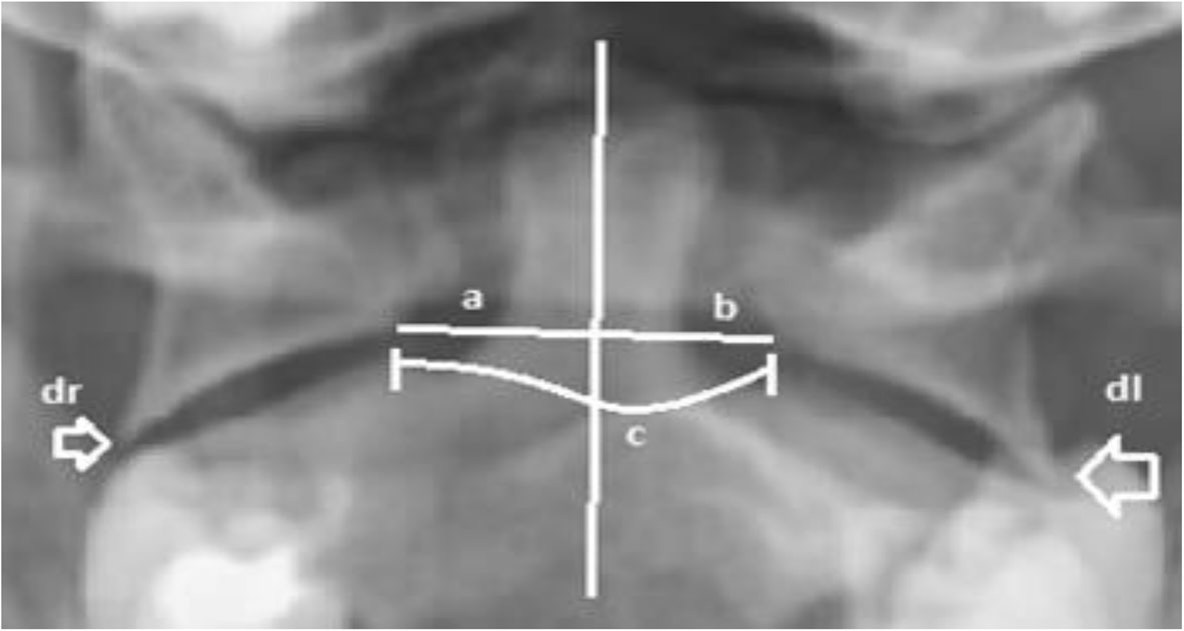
Open Mouth Lateral bending cervical spine radiographs with measures recorded. a midline of Dens to right lateral mass; b midline of Dens to left lateral mass; c width between lateral mass; dr: Right lateral mass step-off; dl: left lateral mass step-off
